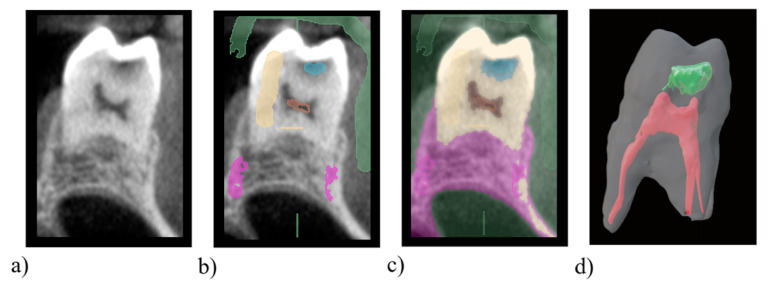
Conception of an educational model with carious process using a free software 3DSlicer. (a) 3D image from a cone beam computed tomography of a maxillary molar presenting a cavity. (b) Attribution of pixel labels for air (green), dentin (yellow), pulp (red), cavity (blue) and bone (purple) using the “paint” tool on the 3D image. (c) Segmentation of the 3D image using the “Grow from seeds” tool. (d) Export of the pulp, cavity and tooth STL models and visualization in the software Blender using transparent labels.
